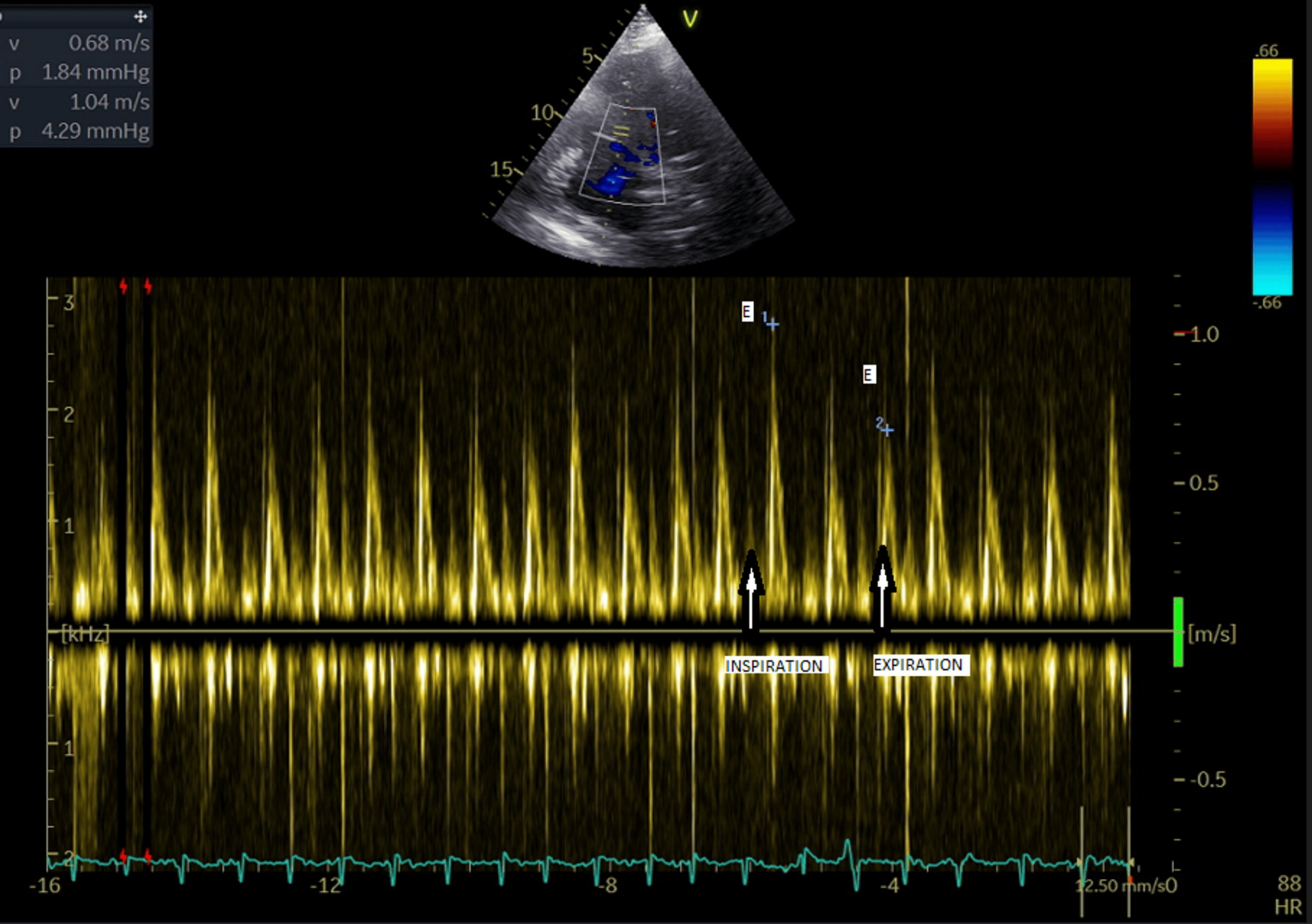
Echocardiography Pulsed Doppler at the level of the tricuspid valve with respiratory variations. This pattern of respiratory variation is frequently associated with constrictive pericarditis.
